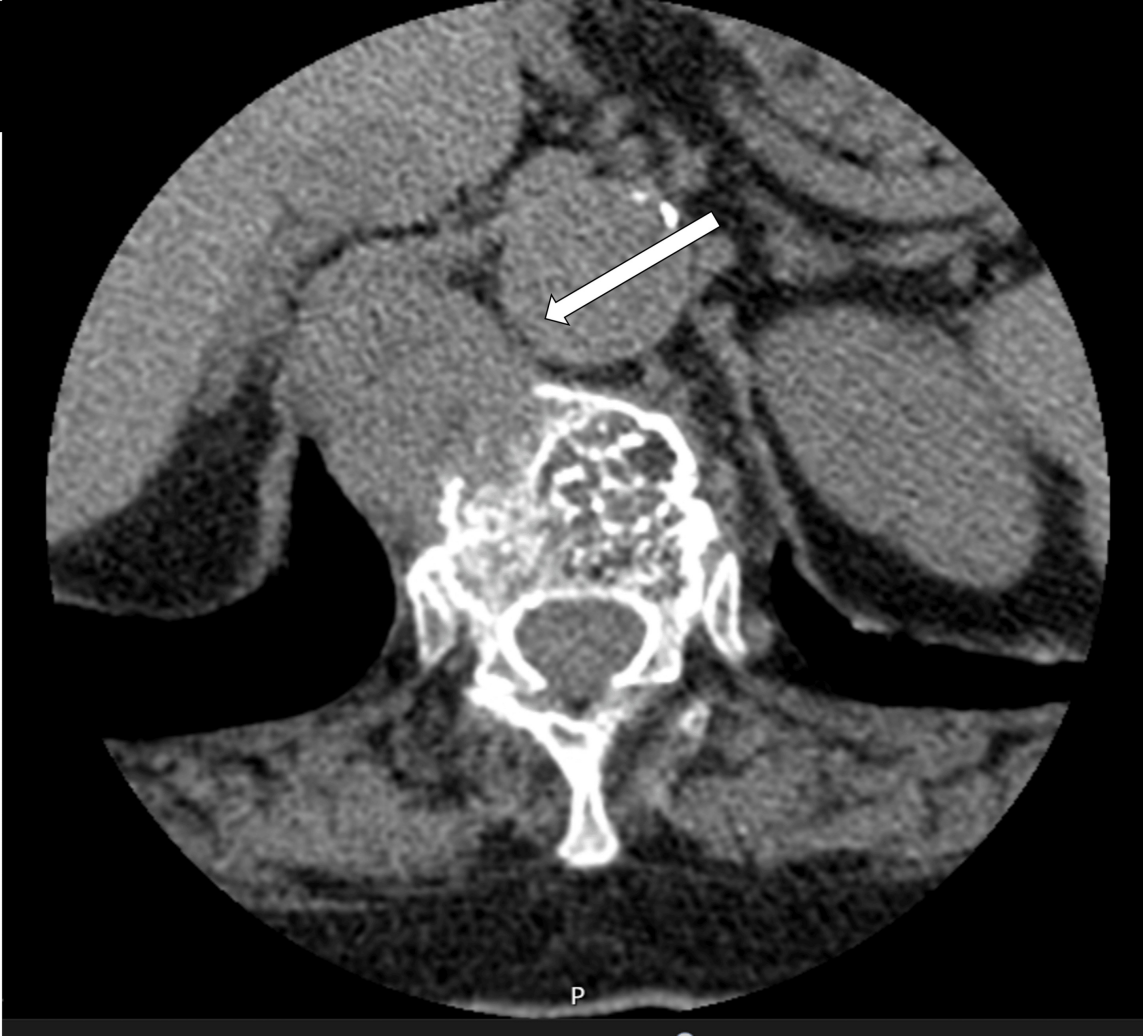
Thoracoabdominopelvic computed tomography scan showing an expansive osteolytic lesion of the D12 vertebral body (white arrow)
